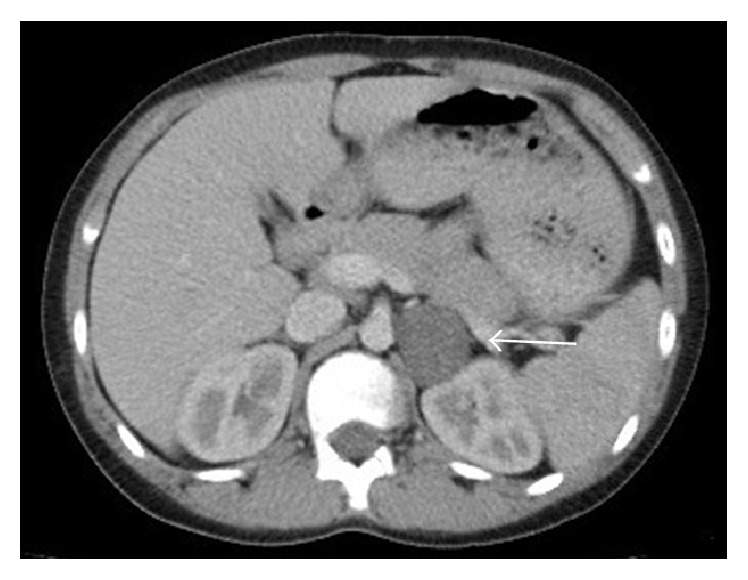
A 32 × 40 mm homogeneous mass (white arrow) in the left adrenal region showing a regular contour on computed tomography.
